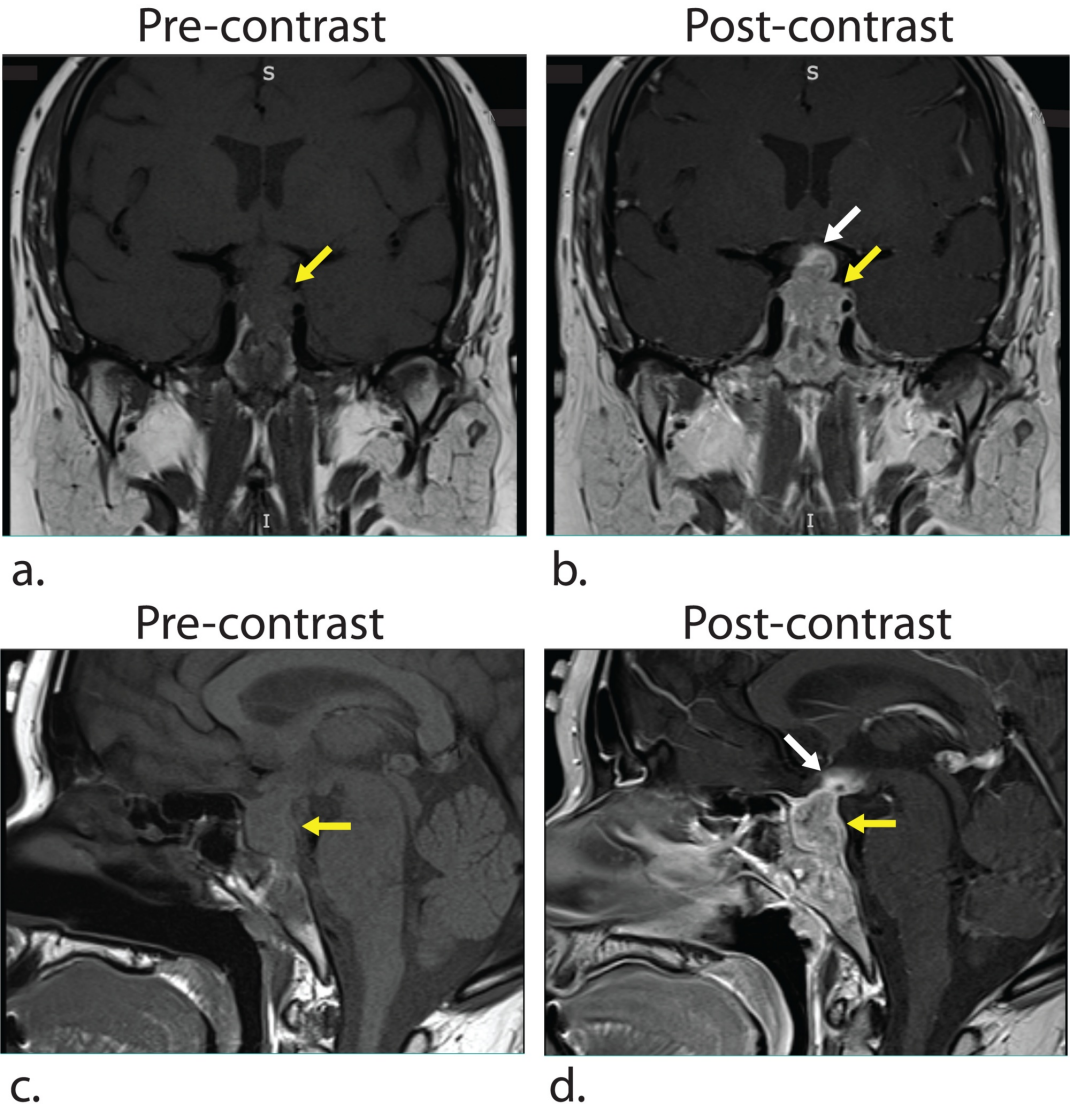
Repeat MRI showed interval enlargement and contrast enhancement of the lesion Pre-contrast T1-weighted coronal (a) and sagittal (c) MRI images demonstrate a 1.5 x 1.6 x 2.4 cm (AP x TR x CC) lesion in the sella turcica (yellow arrows) with supra-sellar extension (white arrows). Post-contrast T1-weighted coronal (b) and sagittal (d) MRI images show contrast enhancement of the lesion. AP, anteroposterior; TR, transverse; CC, craniocaudal.
